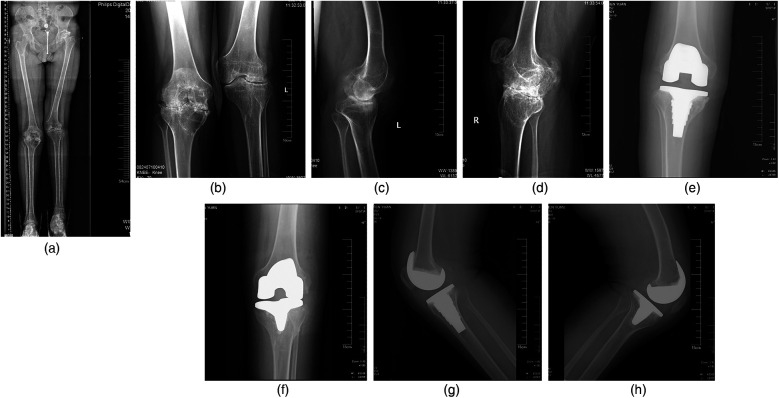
Radiographs of a 28-year-old male patient with severe haemophilic arthropathy. Preoperative x-rays showed extensive destruction of an articular surface, narrowing of the joint space, and a square patella. All operations are performed using mechanical alignment methods. (a) Preoperative anteroposterior radiographs of lower extremity length; (b) preoperative anteroposterior radiograph; (c,d) preoperative lateral radiograph of affected knee joint; (e,f) postoperative coronal knee radiographs; (g,h) postoperative sagittal knee radiographs.
